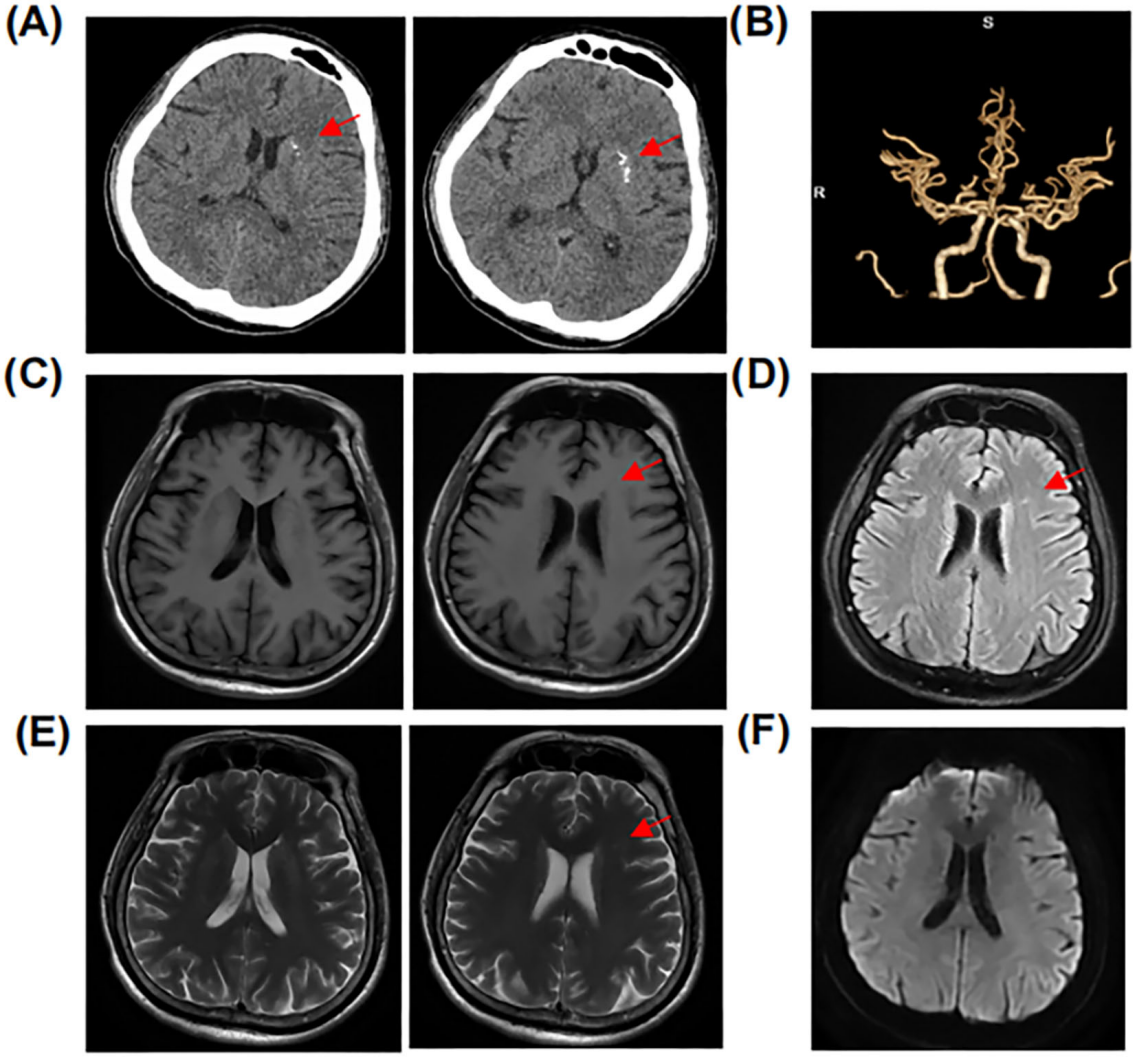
Cranial computed tomography (CT) and brain magnetic resonance imaging (MRI) scans of the patient. (A) Calcification in the left basal region. (B) Head computed tomography angiography (CTA) was unremarkable. (C-F) Brain magnetic resonance imaging (MRI) revealed a few punctate abnormal signals adjacent to the lateral ventricles and the sulci, fissures, and cisterns of the brain were widened.
